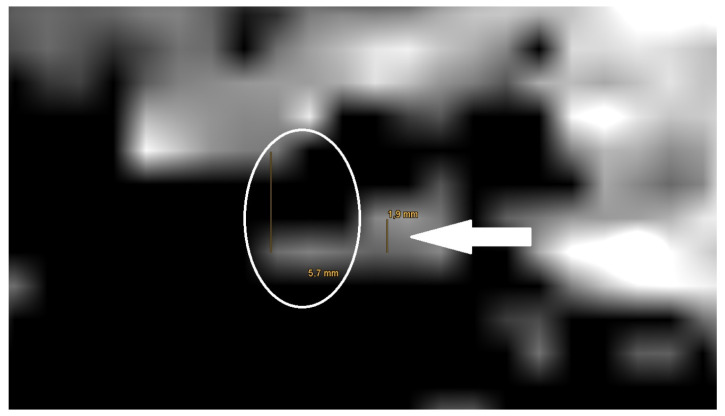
Magnification of the artifact of a mesh on the right side of the lateral skull base in a non-EPI DWI sequence, with measurement (white arrow).
